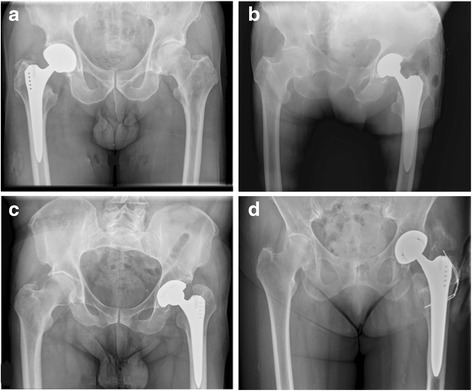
One year follow-up standard radiographic control, examples of heterotopic ossification according to Brooker grade 1 (a), grade 2 (b), grade 3 (c) and grade 4 (d)
